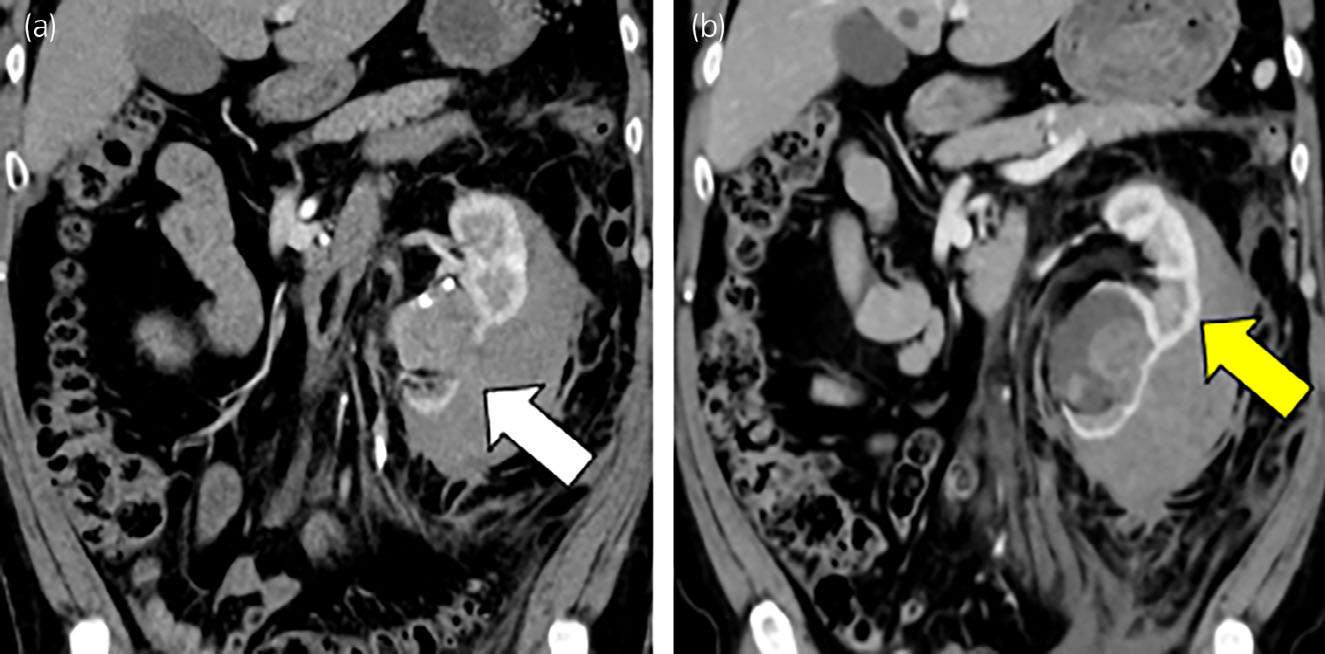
(a) Abdominal and pelvic enhanced CT in coronal view showing a rupture of the lower half of the left renal parenchyma with perirenal hematoma (white arrow). (b) The upper left half of the kidney is normal (yellow arrow).
